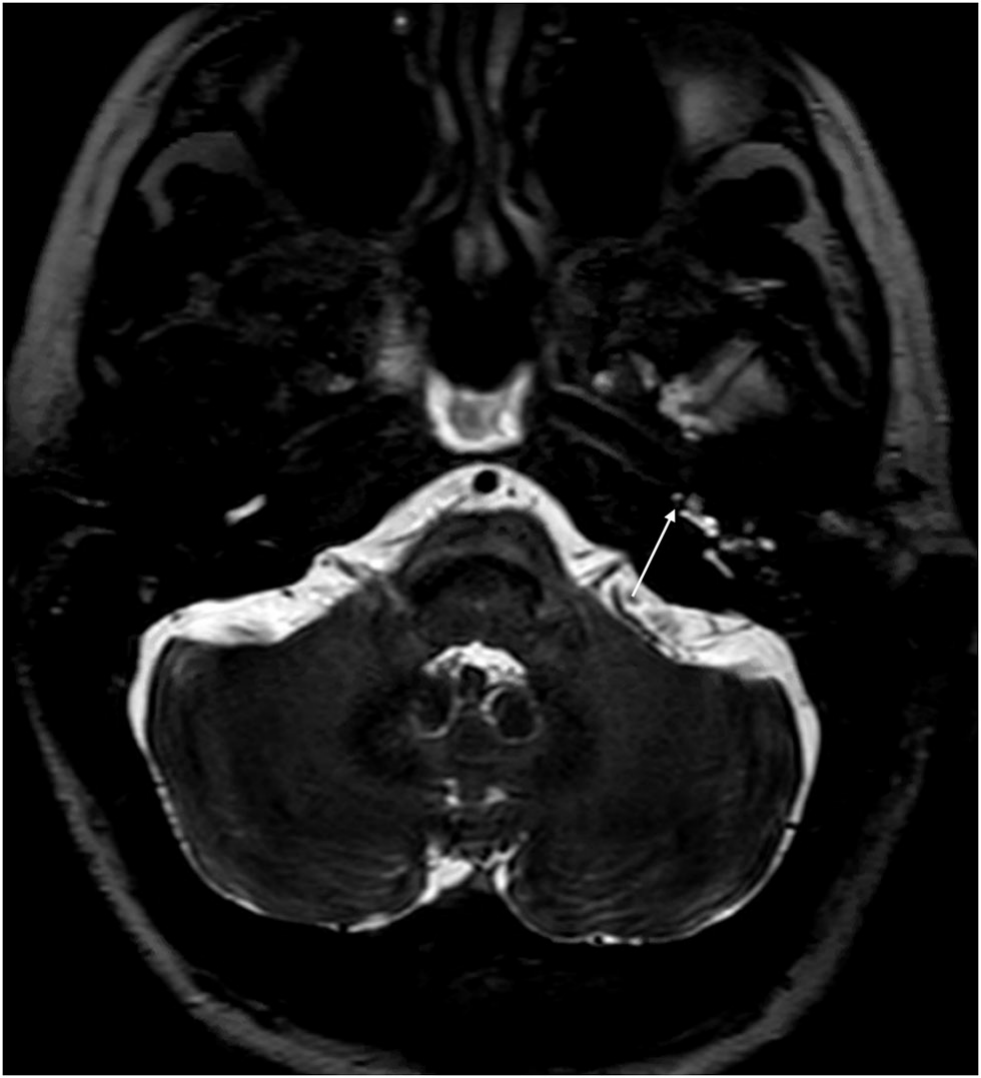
CISS sequence MRI of the same patient as Figure 5 1 day after blood patch procedure. There is a small amount of air in the distal basal turn of the cochlea (arrow).
